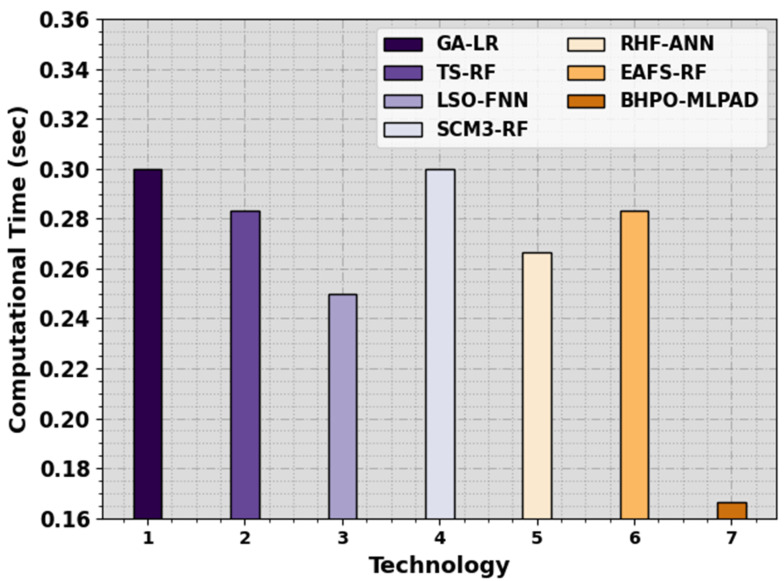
CT outcome of the BHPO-MLPAD approach with other methodologies.
